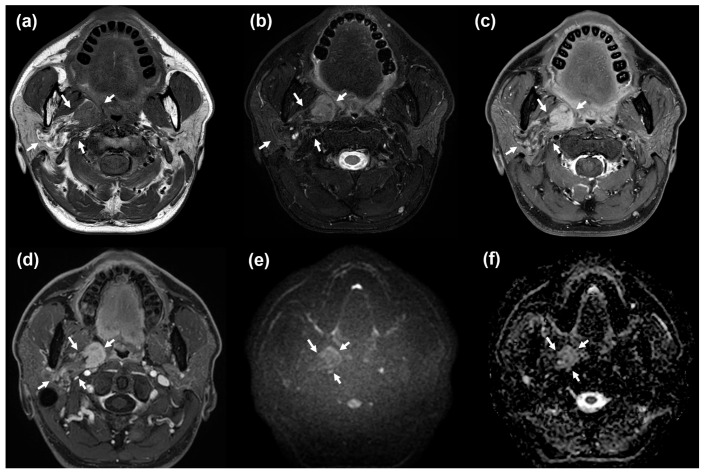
MRI findings. (a) Axial T1-weighted image shows a fat-containing mass centered in the right parotid gland, arising from the deep lobe with medial exophytic extension into the right parapharyngeal space, and a discrete solid portion along the medial aspect (arrows). The lobulated mass is clearly demarcated from the adjacent parapharyngeal fat plane. (b) Axial fat-suppressed T2-weighted image shows tiny hyperintense cystic foci within the lesion. (c,d) Axial fat-suppressed contrast-enhanced T1-weighted images at different levels show heterogeneous enhancement of the solid portion (arrows). (e,f) Axial diffusion-weighted image (DWI) and the corresponding apparent diffusion coefficient (ADC) map demonstrate mild diffusion restriction in the solid portion (arrows).
